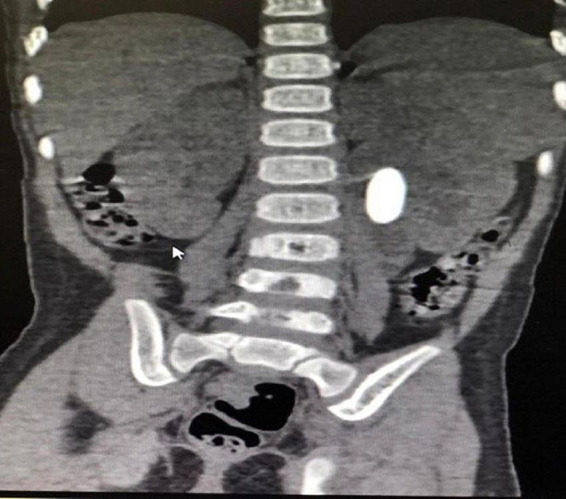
CT KUB showing hydronephrotic left kidney (PD-13.1 mm), with 2.4 cm × 1.1 cm oval calculus, normal ureter.
